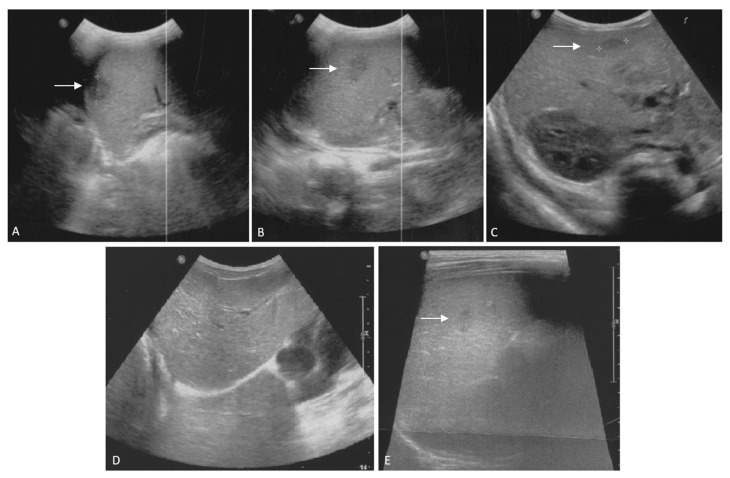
Liver and spleen ultrasound scans of the patient on admission (A–C) and during follow-up (D,E) in a 4-year-old immunocompetent child with atypical bartonellosis. Admission. (A,B): enlarged inhomogeneous spleen with multiple hypoechoic lesions (A): ∅ 15 mm; (B): ∅ 17 mm). (C): liver appears of increased echogenicity, with multiple hypoechoic lesions; the arrow indicates the larger one (∅ 15 mm) in hepatic segment V. Enlargement of hilar hepatic lymphnodes can be appreciated. After one month from admission. (D,E): abdominal ultrasound shows the disappearance of the hepatic lesions (D) and the persistence of two hypoechoic spleen lesions, in decline (E): largest lesion, ∅ 7 mm, previously ∅ 17 mm as shown in (B).
